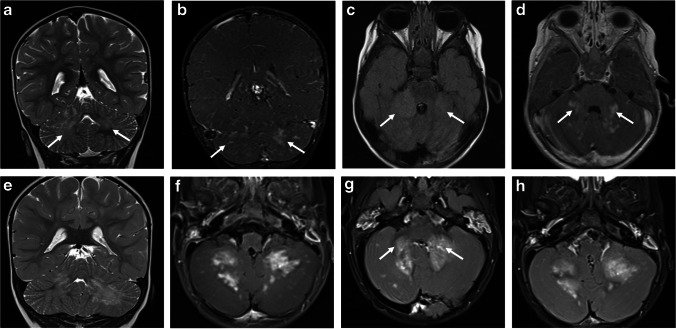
Cerebellar involvement in two children with myelin oligodendrocyte glycoprotein antibody-associated disease and acute disseminated encephalomyelitis. Magnetic resonance images of the brain obtained from a 20-month-old girl (a-d) and from a 15-month-old girl (e-h). a Coronal T2-weighted and (b) coronal fat-suppressed contrast-enhanced T1-weighted images show ill-defined T2-hyperintensities involving asymmetrically the bilateral cerebellar lobes (*arrows* in a), associated with nodular and patchy enhancement (*arrows* in b). c Axial fluid-attenuated inversion recovery (FLAIR) and (d) axial contrast-enhanced T1-weighted images show the lesions involving the bilateral middle cerebellar peduncles (*arrows* in c and d). e Coronal T2-weighted, (f) axial fat-suppressed contrast-enhanced T1-weighted, and (g, h) axial fat-suppressed contrast-enhanced FLAIR images show bilateral lesions involving the cerebellar lobes (e) and middle cerebellar peduncles (*arrows* in g), associated with avid confluent nodular enhancement (f-h)
